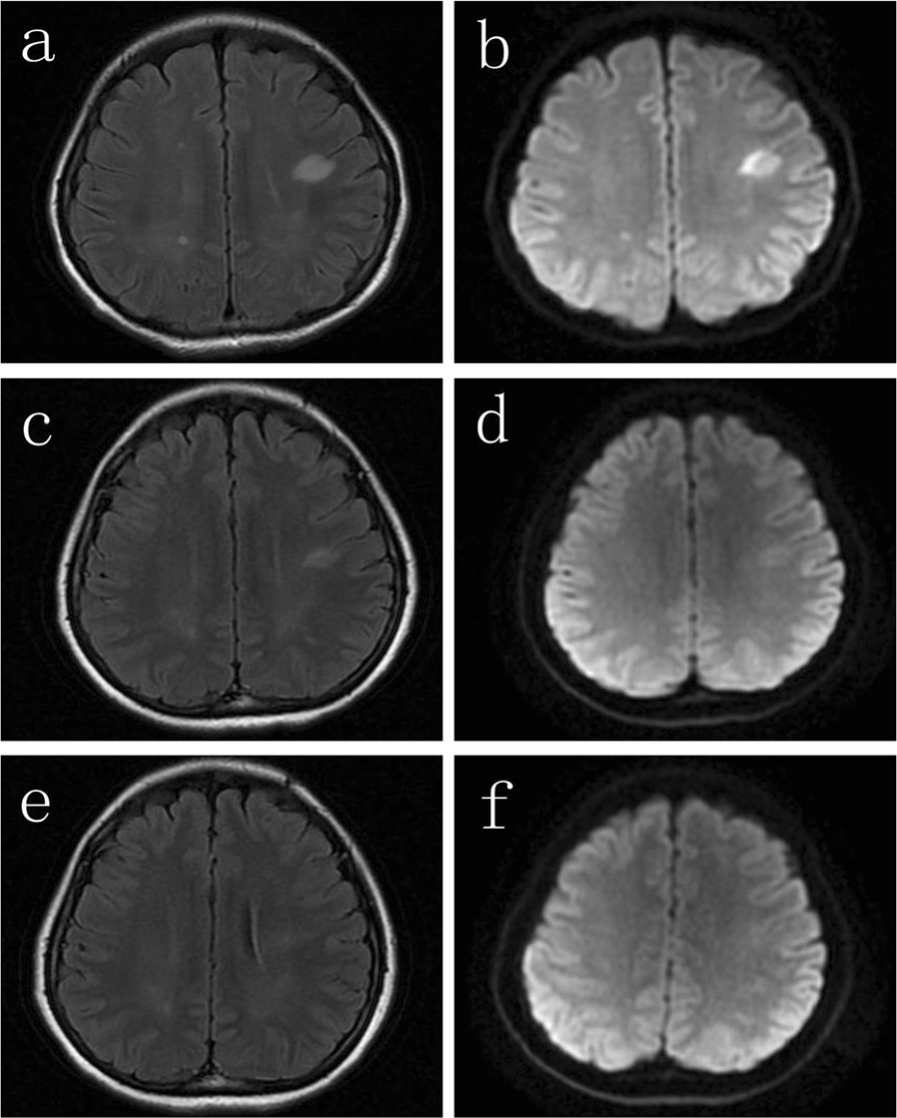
Serial MRI findings: hyperintense lesions on FLAIR and DWI in bilateral subcortical white matter before plasma exchange (a, b); partial resolution of the lesions on FLAIR and DWI 1 month after plasma exchange (c, d); full resolution of the lesions on FLAIR and DWI 2 months after plasma exchange (e, f)
